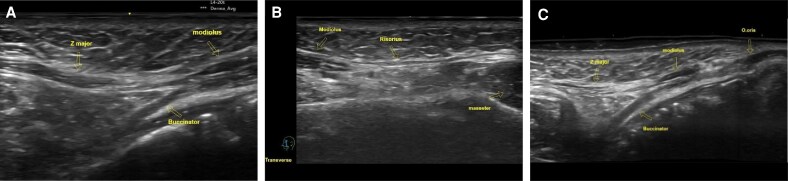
(A) Ultrasound image with the probe in transverse, the zygomaticus major and the buccinator muscles are shown as they insert into the modiolus. (B) Ultrasound image with the probe held in transverse showing a thin hypoechoic band. The risorius muscle arises from the anterior border of the masseter muscle with insertion into the modiolus. (C) A panoramic view with the probe in transverse showcases the ultrasonic location of the modiolus based on the convergence of zygomaticus major, buccinator, and the orbicularis oris muscle.
